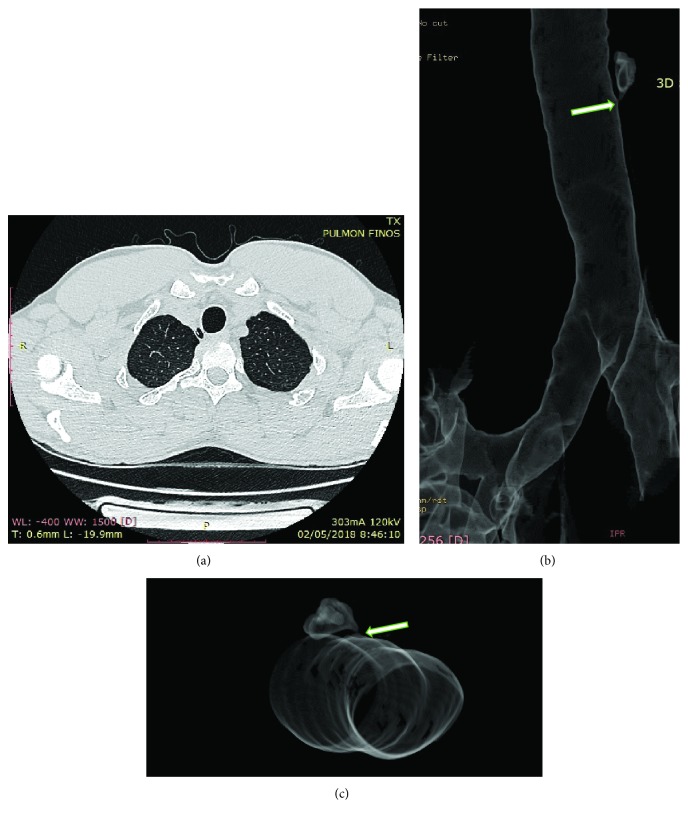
The axial CT (a) shows right paratracheal air cysts, measuring 15 mm in the right posterolateral side of the trachea at the level of C6. On 3D reconstruction (b, c), the white arrow shows communicating channel with the tracheal lumen.
